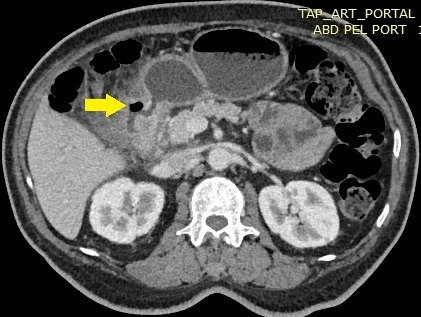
CT scan showing the duodenal diverticulitis
